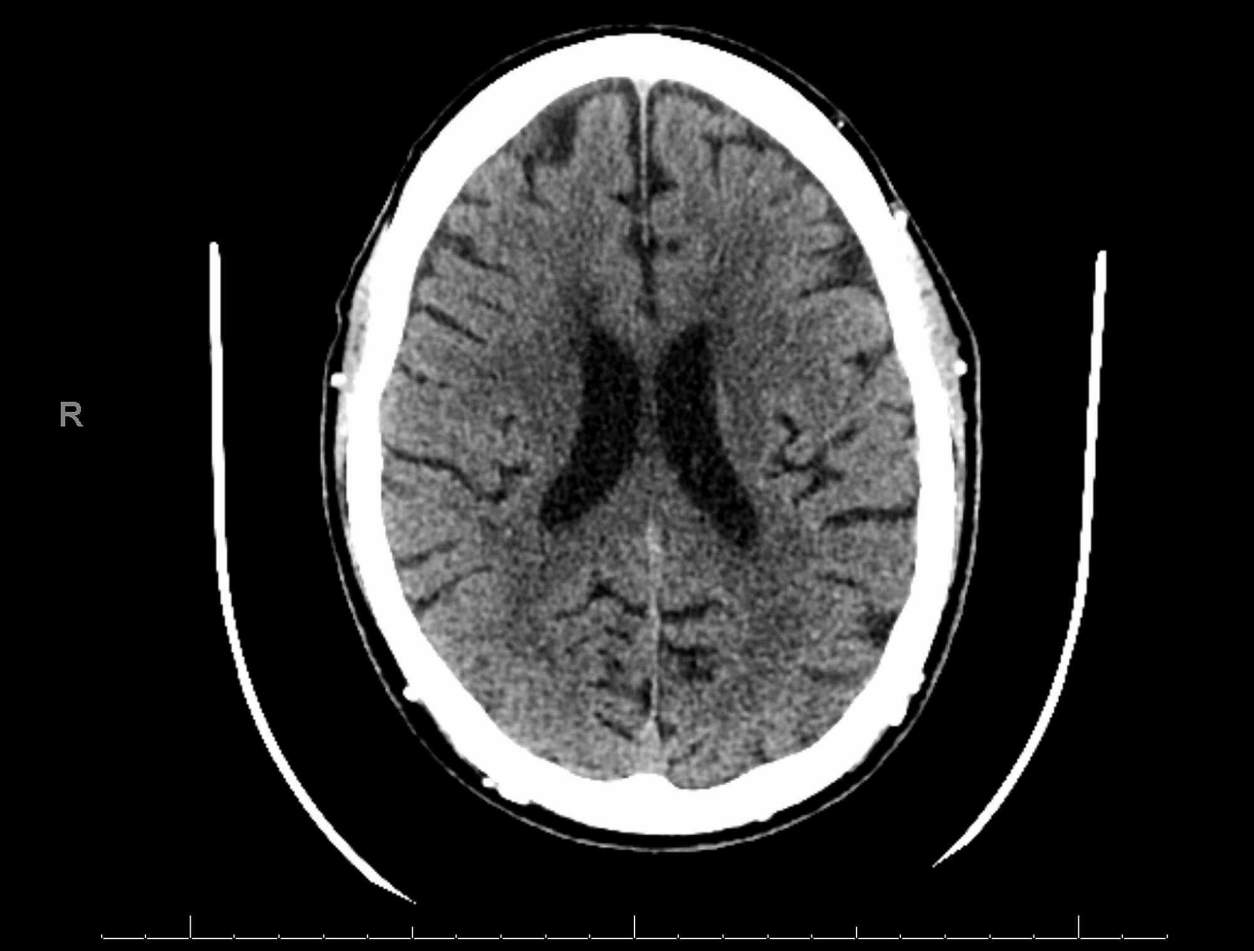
CT of the head revealing no acute intracranial hemorrhage, midline shift, mass effect, or extra-axial fluid collection. The gray-white differentiation is preserved. Brain volume and ventricular system are within normal limits for age
